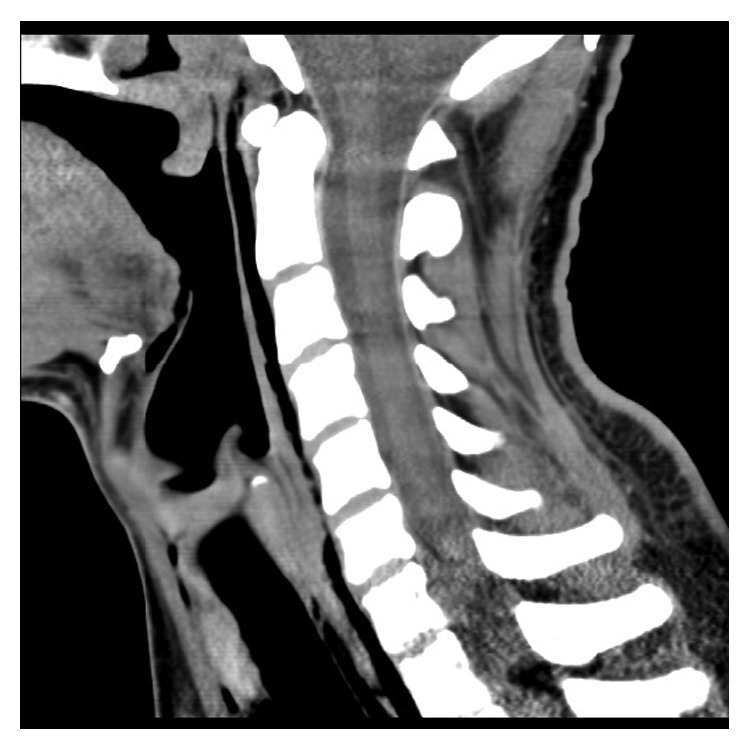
Sagittal CT showing retropharyngeal emphysema extending to the skull base.
